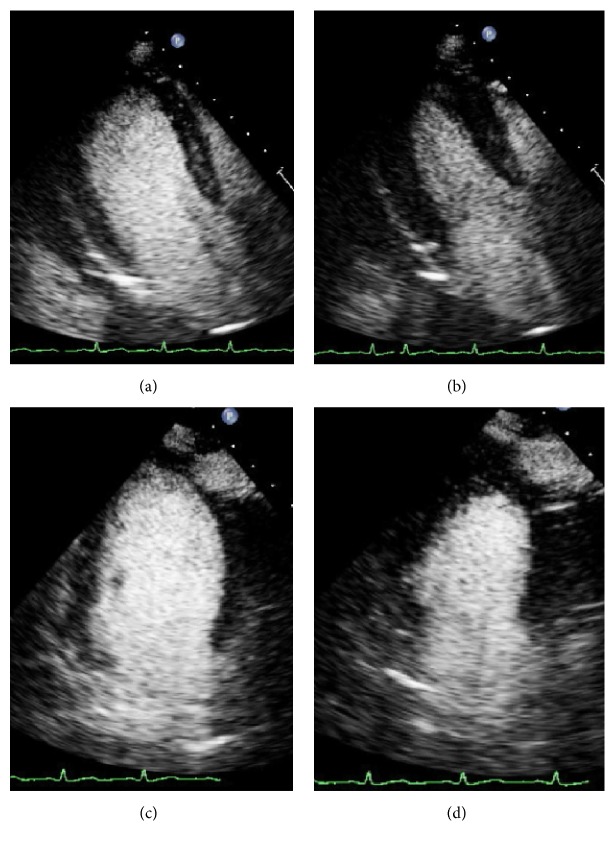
(a) Contrast transthoracic echocardiogram 3-chamber view of left ventricular cavity at end-diastole. (b) 3-chamber view at end-systole showing global contraction. (c, d) 4-chamber view of left ventricular cavity at end-diastole and end-systole, respectively.
